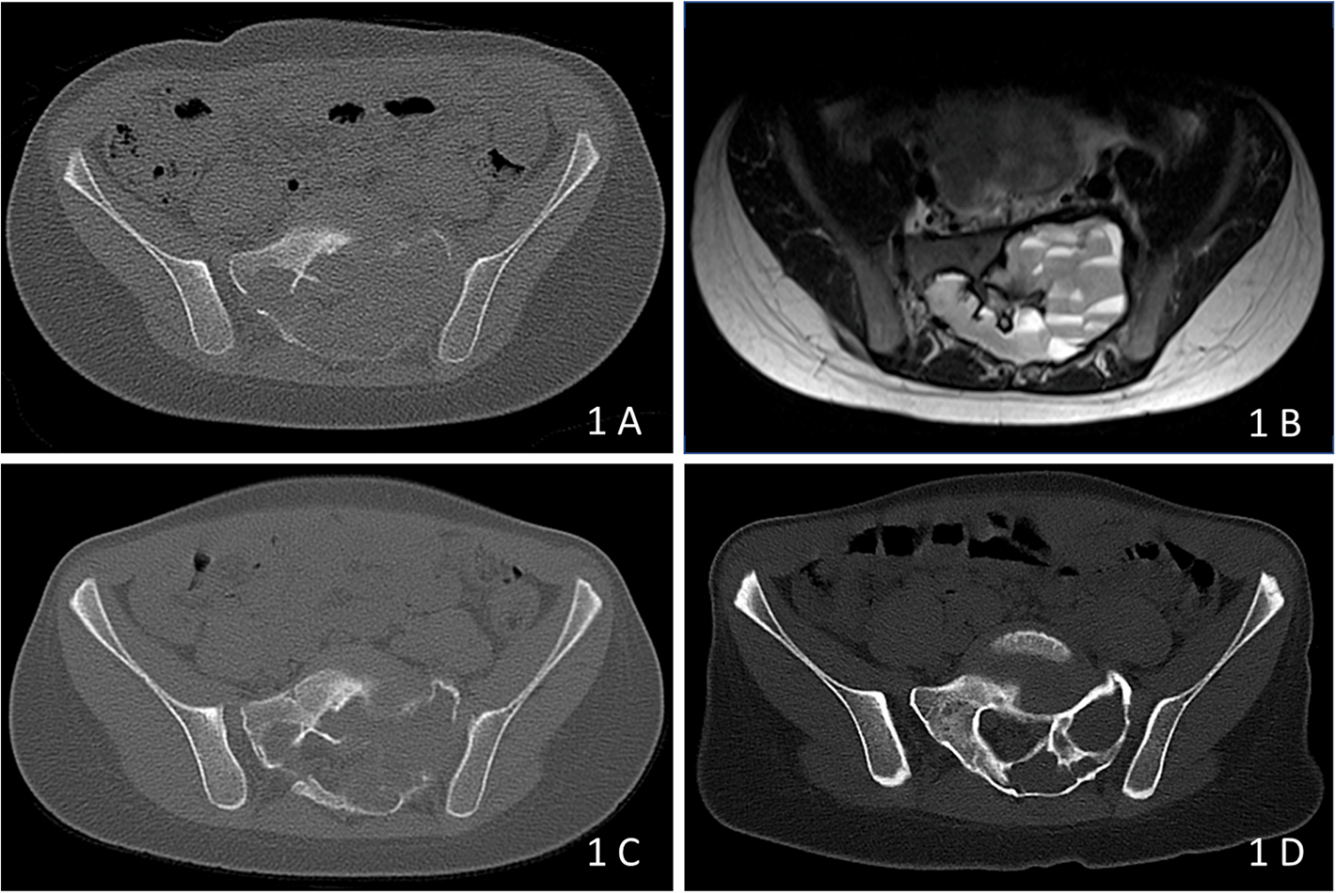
a, b: CT-scan (A) showing the massive destruction of the left and also in parts the right sacrum at the levels S1 and S2. The blood filling of the cyst is clearly visible as fluid-fluid levels in the T2-weighted MRI image (b). c: CT-scan 2 months after initiation of treatment showing increasing bone density at the margins of the lesion. d: CT-scan one after 1 year of treatment proofing the total bone restoration in the right and the impressive gain of bone at the left sacrum
